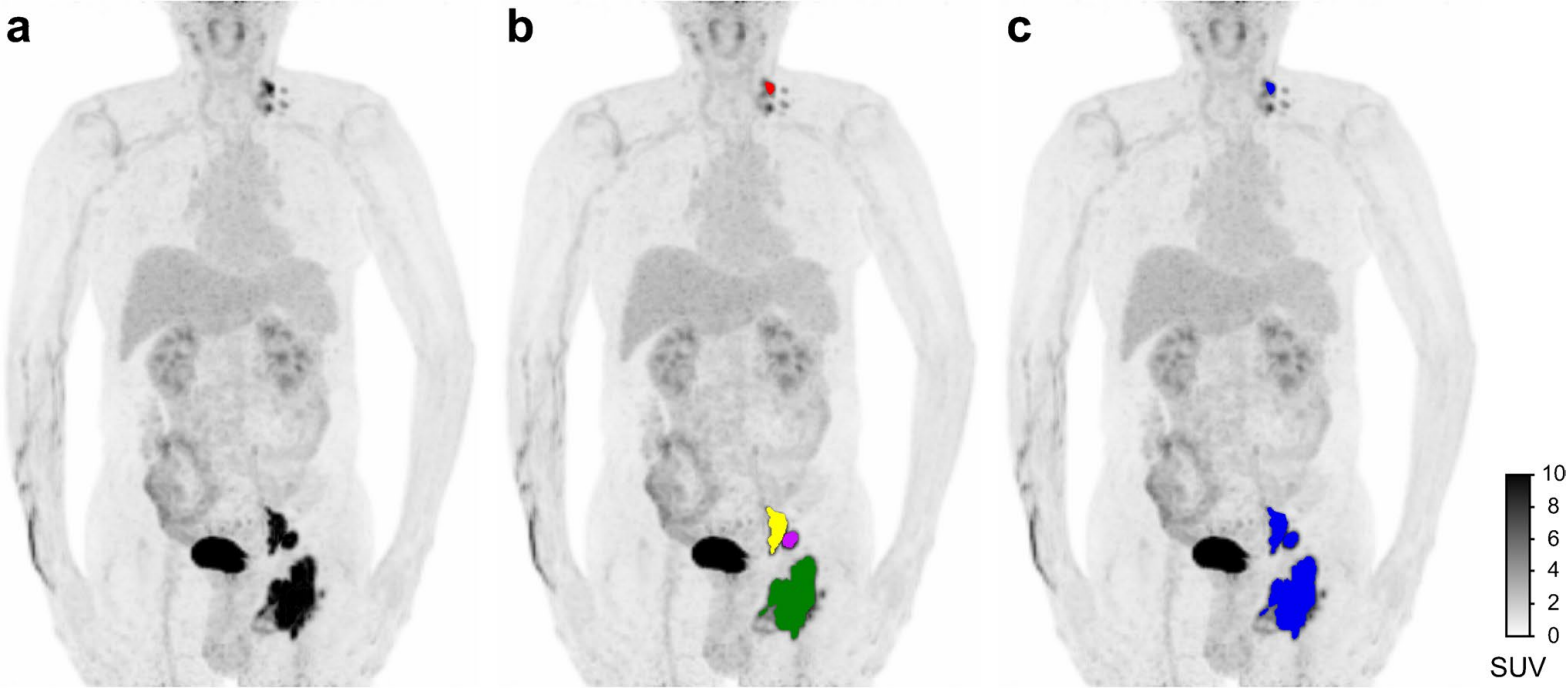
Representative [^18^F]FDG PET images of metabolic bulk volume (MBV) and total metabolic tumor volume (TMTV) delineation. a Anterior maximum intensity projection image. b The VOIs of cervical (red), iliac (yellow and purple), and inguinal (green) lymph nodes were semiautomatically delineated using the 41% SUVmax threshold method. The VOI of inguinal lymph node (green) represents the MBV. c TMTV (blue) was constructed using the “save all in one” function in LIFEx
